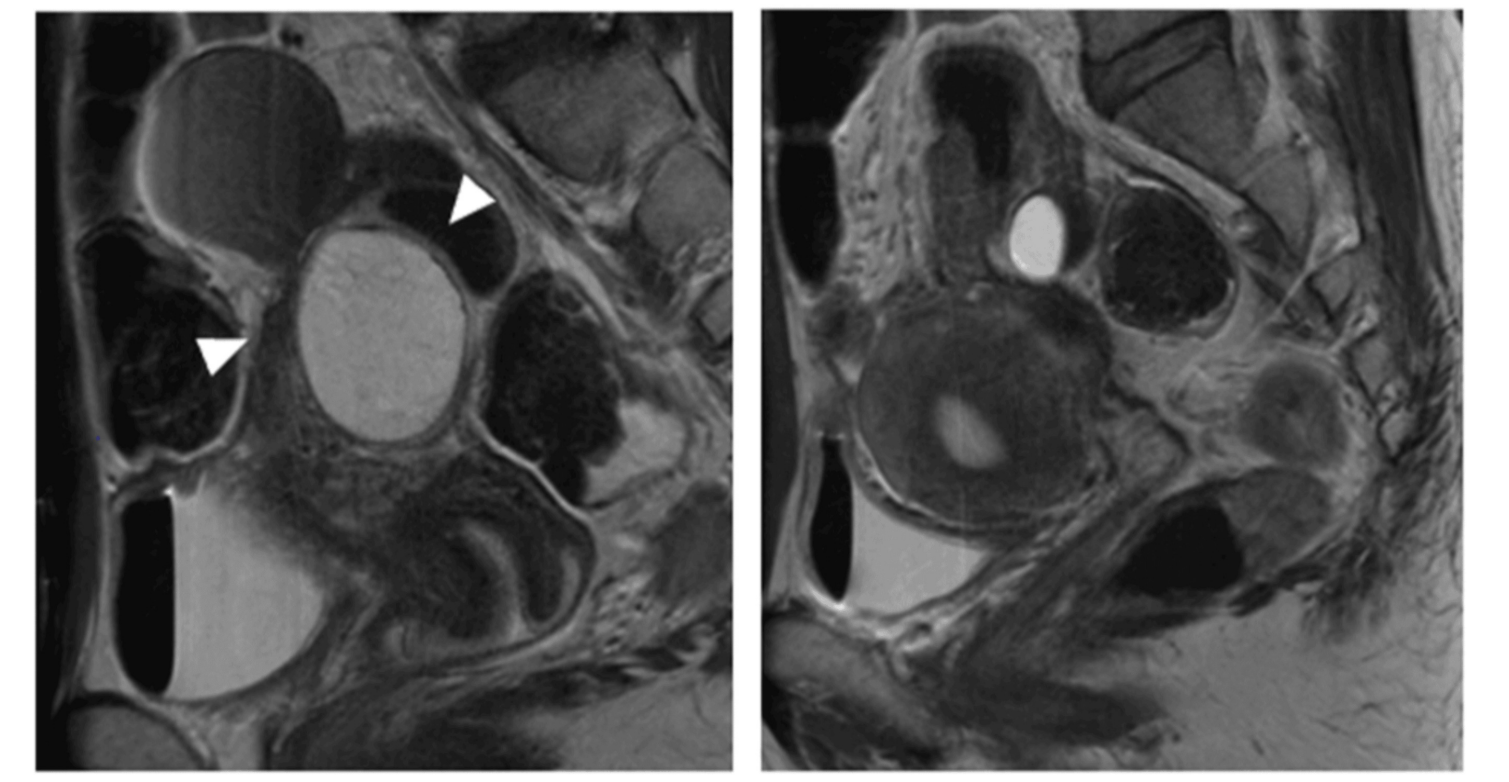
MRI before the third surgery. (Left) Right endometrioma is indicated by white triangles. (Right) Uterine axis was displaced to the left.
